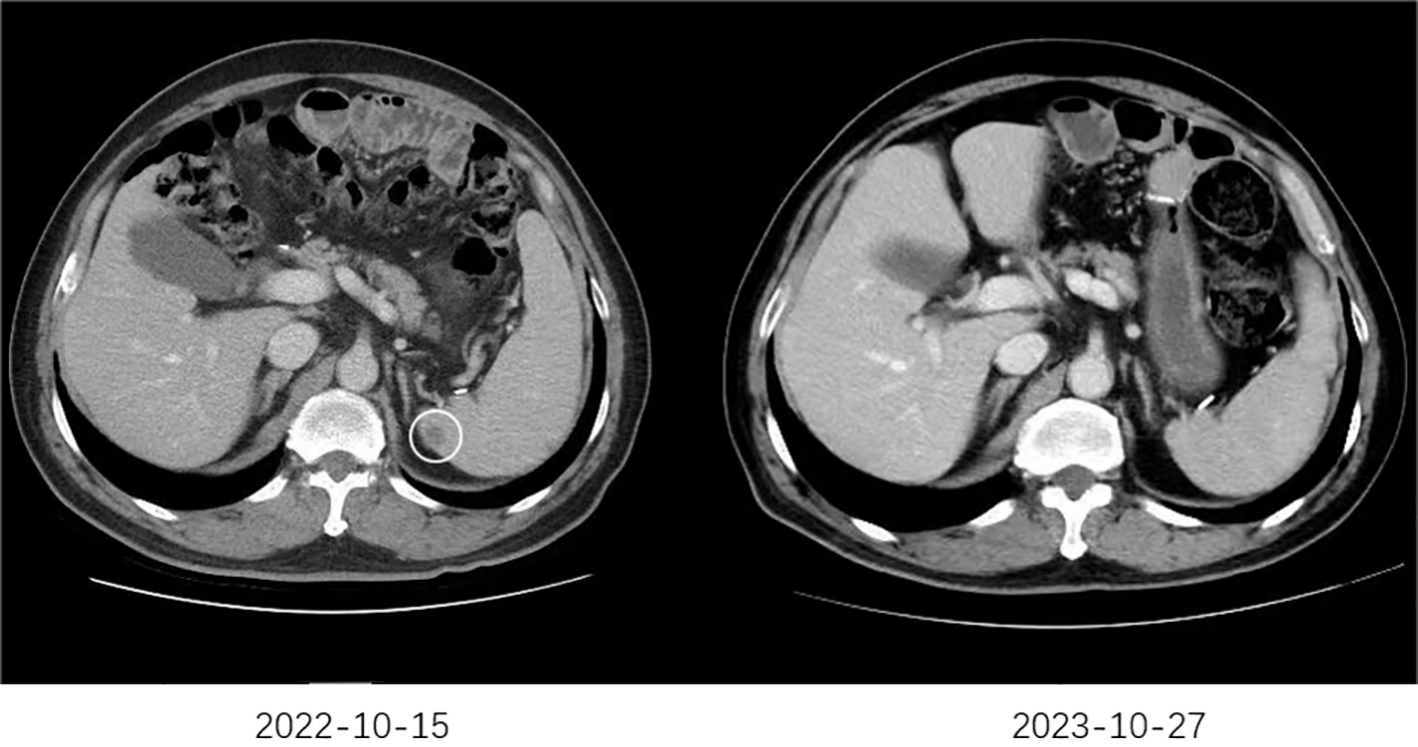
The typical CT images of a CR patient. The CT scan performed on October 15, 2022, revealed newly developed splenic metastases. Subsequently, the patient began first-line treatment on October 18, 2022, consisting of six cycles of sintilimab combined with trastuzumab and chemotherapy. Maintenance therapy with sintilimab, trastuzumab, and chemotherapy was continued thereafter. A follow-up CT scan on October 27, 2023, demonstrated the disappearance of the splenic metastases.
